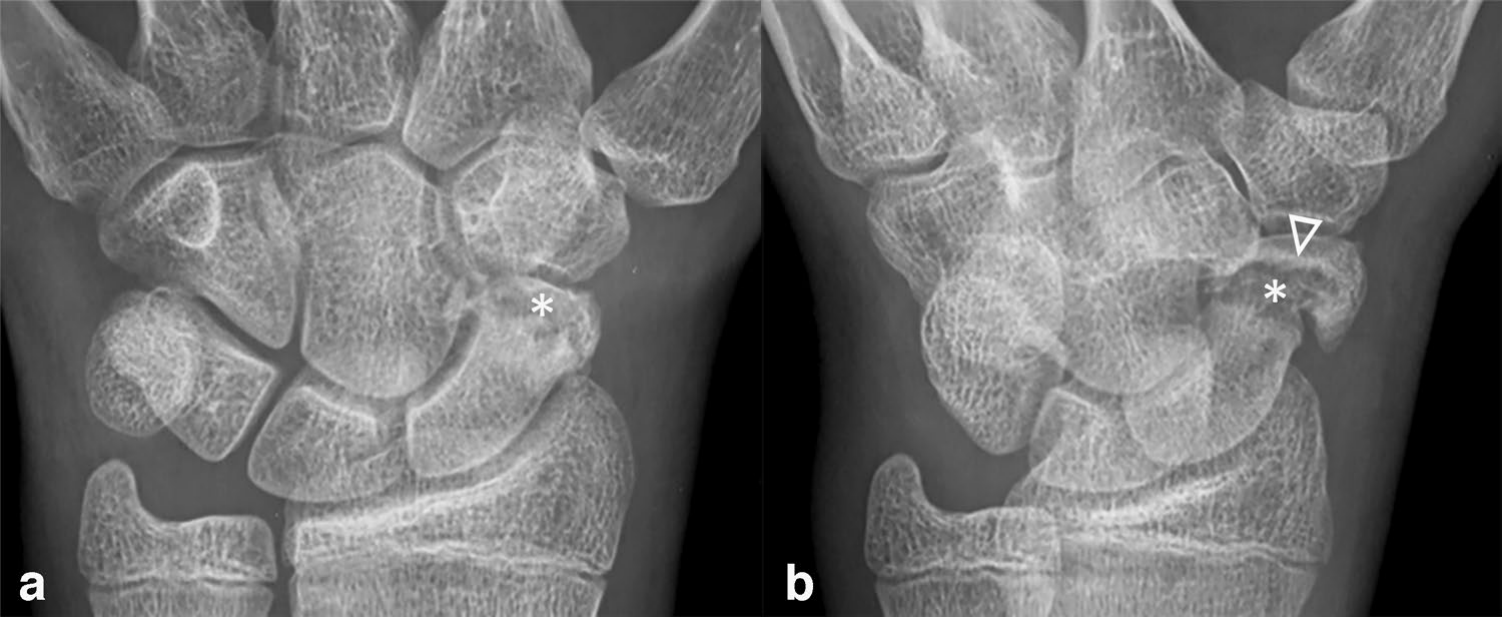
Perifracture findings in a skeletally immature 15-year-old boy. PA (a) and oblique (b) radiographic views show a fracture of the distal pole with lobulated resorption (asterisks) and perifracture radiodensity (triangle). The patient underwent ORIF with an iliac crest autograft
